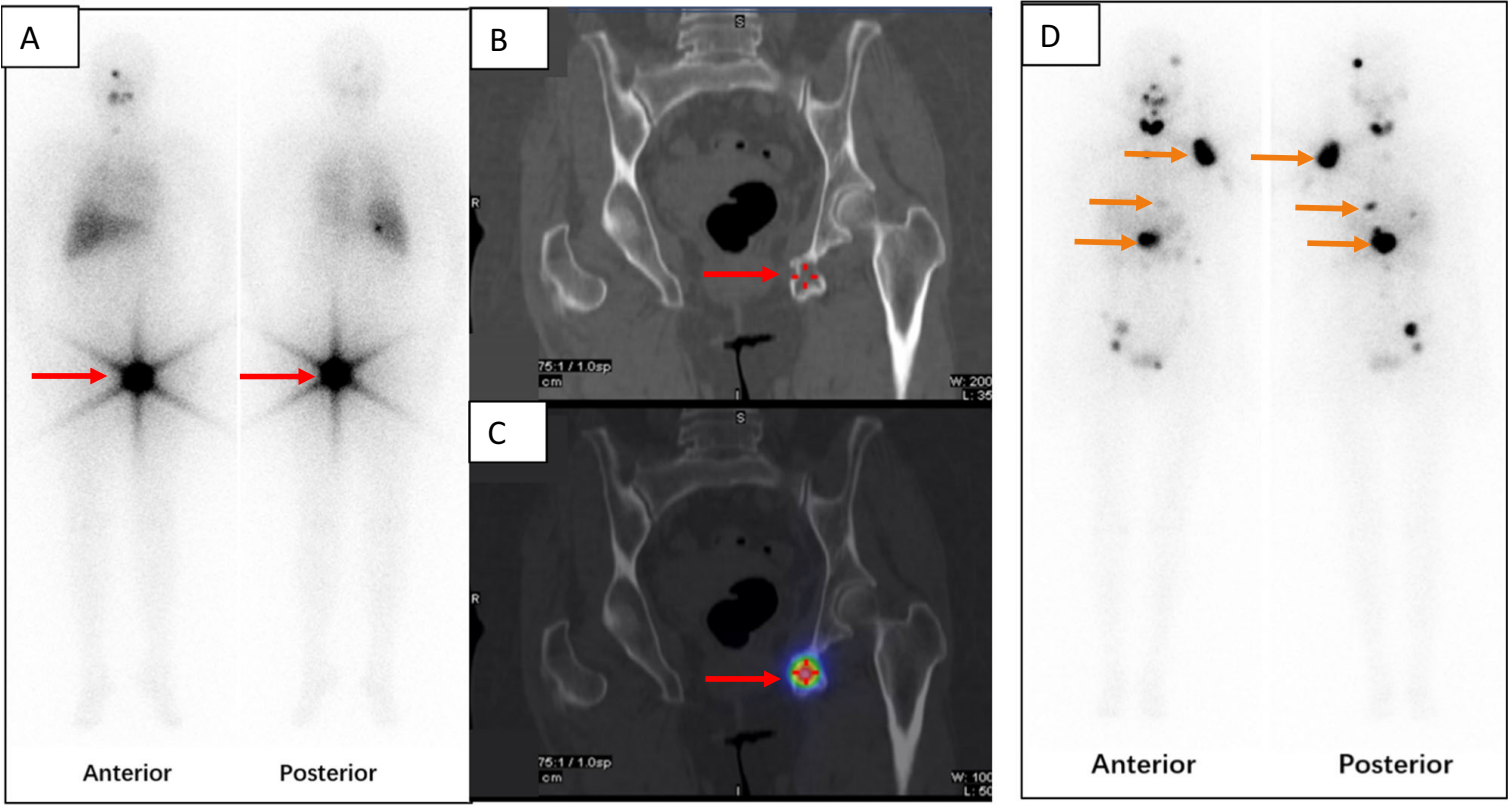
The post-therapeutic ^131^I whole-body scans of patient (No. 2 in Table 1) in anterior and posterior views (A) as well as CT (B) and SPECT/CT (C) of pelvic bone were shown. Red arrows indicated metastases. A post-therapeutic ^131^I whole-body scans of another patient (No. 3 in Table 1) in anterior and posterior views (D) were demonstrated. Orange arrows indicated metastases
